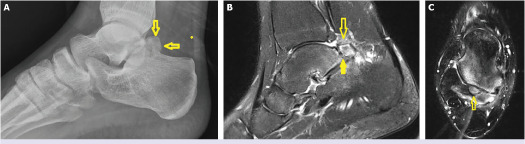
Fifteen-year-old boy with posterior ankle impingement syndrome. (A) On the lateral of the foot radiogram, the os trigonum with a sclerotic signal close to 1 cm is seen in the posterior neighborhood of the talus (arrow). (B, C) There is no connection between the os trigonum and the talus in the fat saturated T2W sagittal and axial plane images. The ossicle, which is the signal of medullary edema on MRI, is seen as a separate bone structure (arrows). In addition to os trigonum edema, talus and soft edema can be selected on MRI
